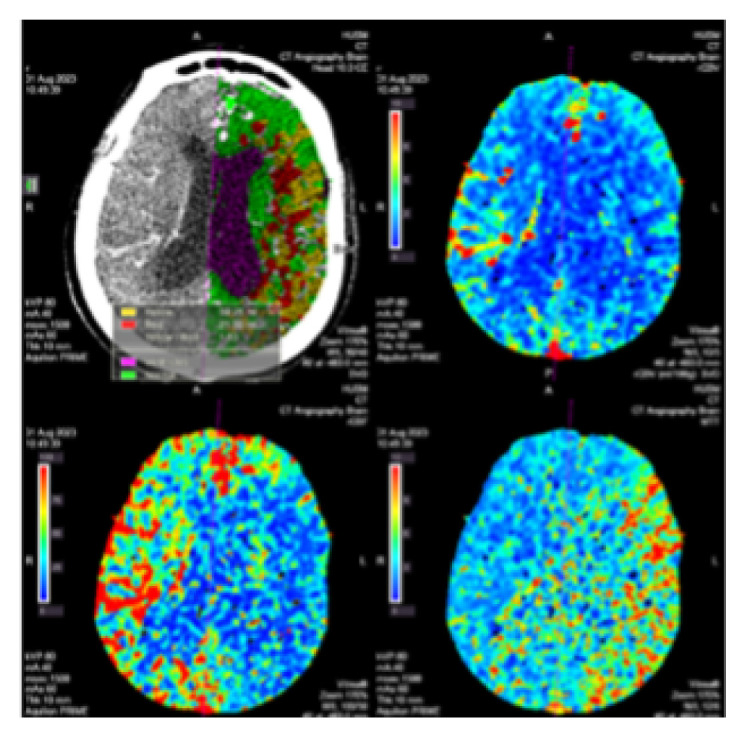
CT Perfusion image of Madam M demonstrating a core infarct surrounded by penumbra in the left M4, M5, and M6 territories. The infarct core volume measures 21.50 mL, with a surrounding penumbra volume of 39.25 mL, yielding a penumbra-to-core ratio of 1.8
